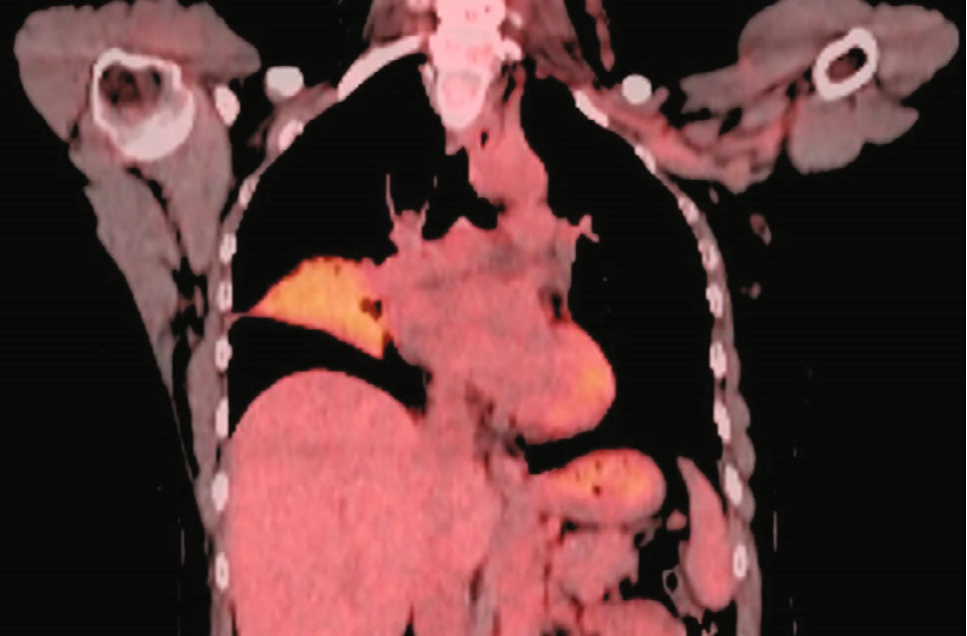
Positron emission tomography scan showing a diffusely avid consolidation (SUV max6.3) in the right middle lobe.
